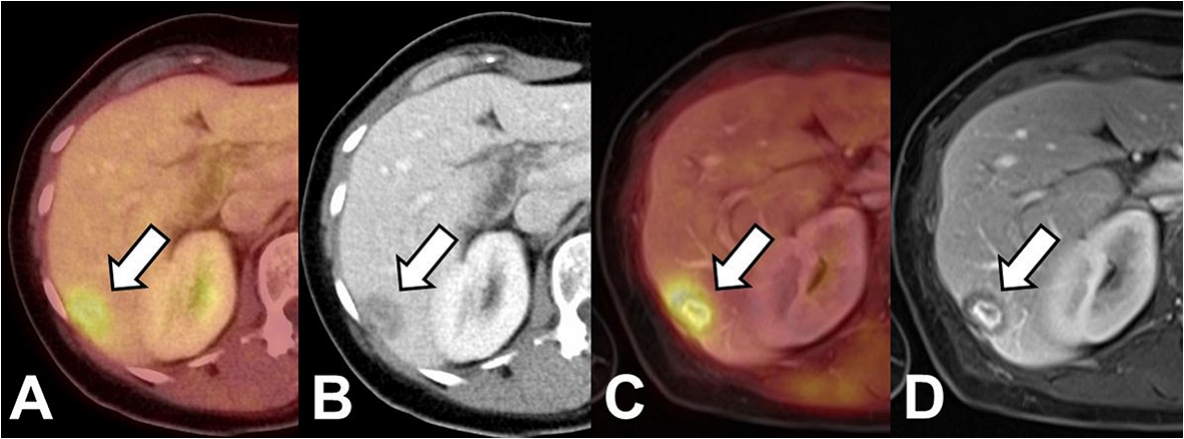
Patient with breast cancer. Both PET/CT (A,B) as well as PET/MRI (C; D; VIBE, portal venous phase) show a lesion with elevated FDG-uptake and ill-defined lesion borders as well as central contrast enhancement as signs of malignancy. Based on these findings the lesion was correctly identified as metastasis in both modalities.
